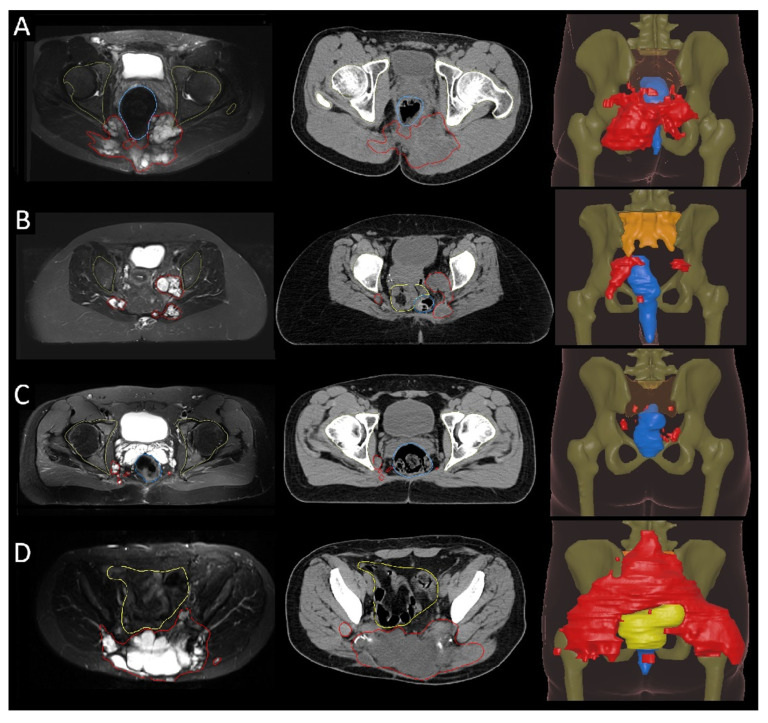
Examples of sacrococcygeal chordoma recurrence in four patients following sacrectomy. Representative axial and sagittal slices from MRI and CT are shown alongside corresponding 3D models. All cases (A–D) demonstrate multifocal soft-tissue recurrence (red); note that residual sacrum (orange) is displayed as transparent in (B,D) for better visualization. Other depicted structures are the rectum (blue), the acetabulum, and femoral heads (olive green), and the bowel (bright yellow).
